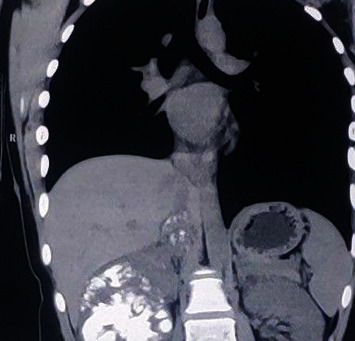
Coronal CT scan showing the involvement of IVC with the tumour thrombus.
